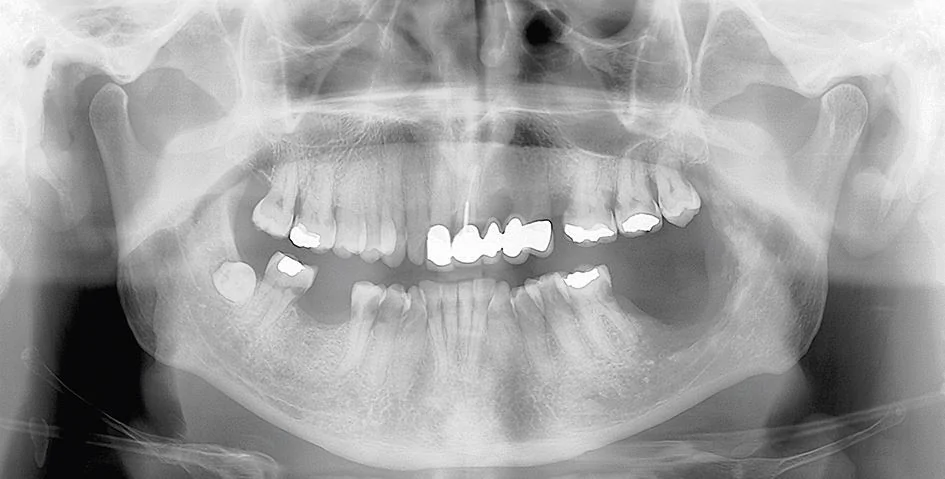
Orthopantomogram showing healing of area of osteoradionecrosis following completion of debridement under general anaesthetic
